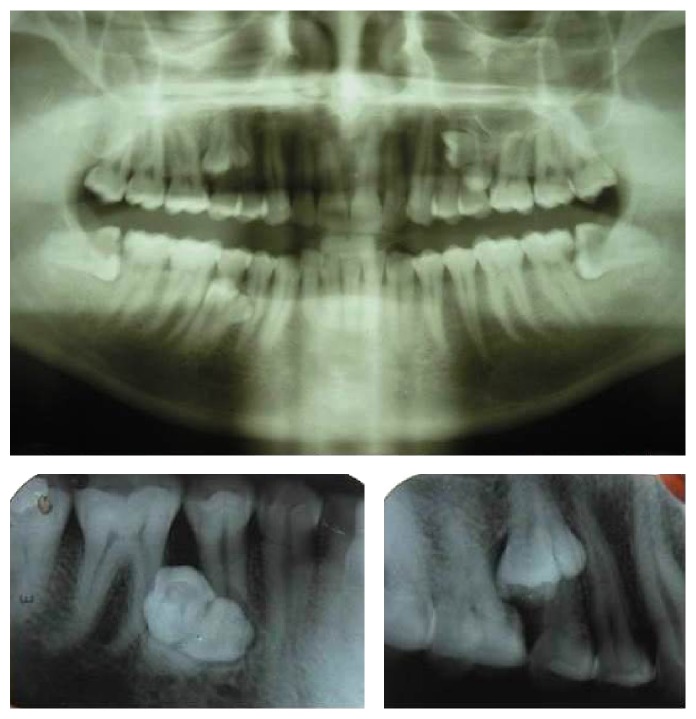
Panoramic radiograph showing the presence of impacted geminated premolars in left maxillary posterior region and right mandibular posterior quadrant. An intraoral periapical radiograph confirms the presence of geminated premolars in the respective regions.
